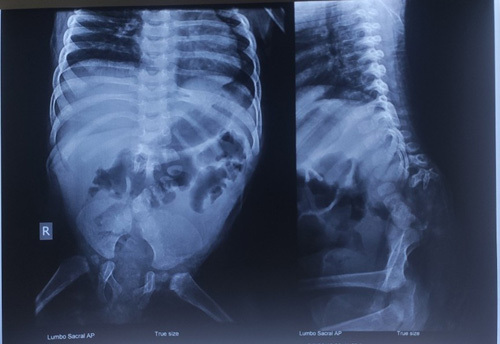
Radiograph of the spine shows incomplete fusion of the iliac bones with a left-sided shift of the lumbar vertebrae. The lowermost lumbar vertebra is articulating with the left iliac bone.
